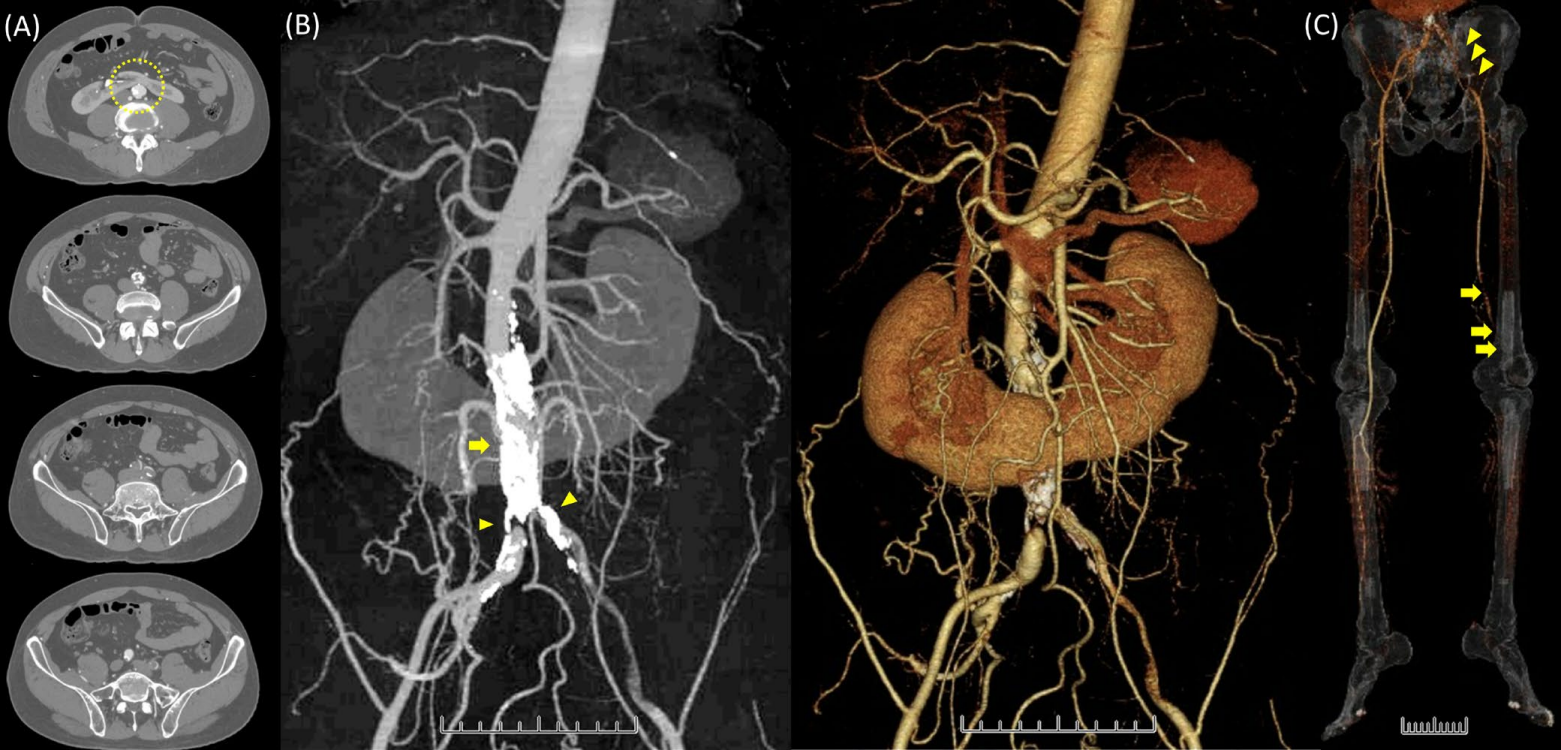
Contrast‐enhanced computed tomography demonstrated: (A) The abdominal aorta running across the horseshoe renal isthmus and the lumbar vertebrae (dotted circle). (B) Severe focal calcification of the aorta adjacent to the horseshoe renal isthmus (arrow), with occlusion of the terminal aorta and the ostia of the bilateral common iliac arteries (arrowheads). (C) Continuous thrombi observed distally (arrowheads) with prolapsed thrombi occluding the popliteal artery (arrow).
